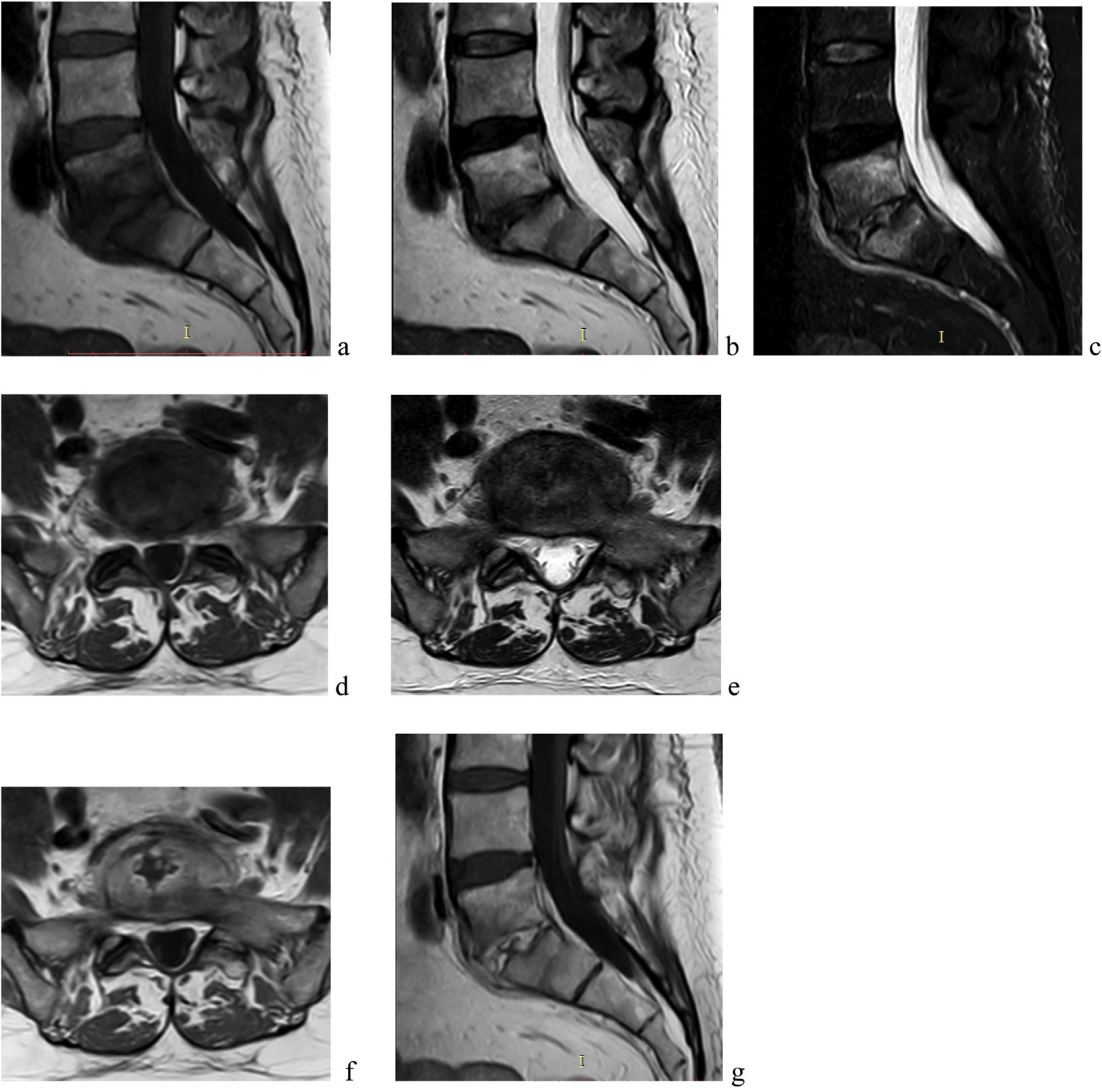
Lumbar spine MRI at time of *Bacillus circulans* isolation.
